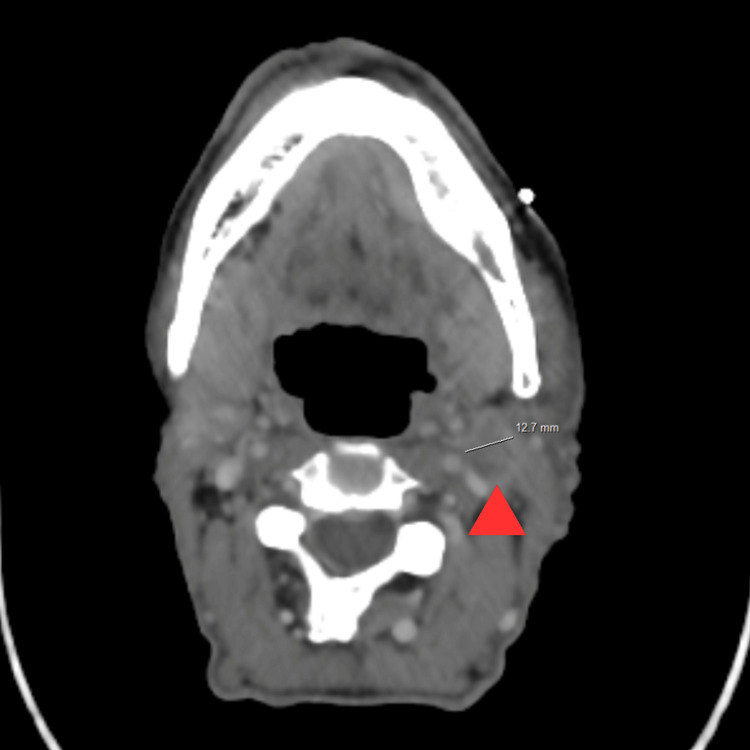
Post-contrast cervical CT in the mediastinal window demonstrates a left cervical lymph node with central necrosis, measuring 13 mm in short axis CT: computed tomography
